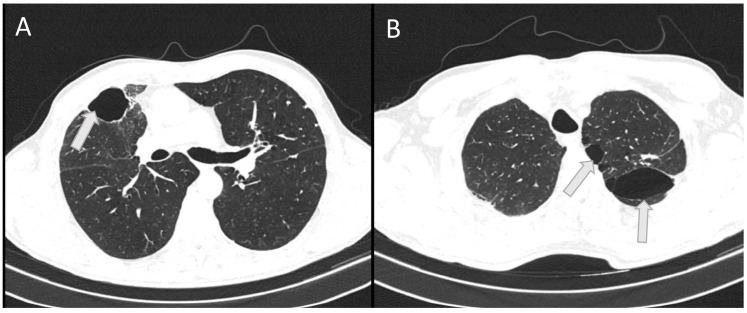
The horizontal CT scan section at the level of the 6th thoracic vertebra (A) and at the level of the 3rd thoracic vertebra (B) showing residual bullous changes (arrows) 6 months after severe chest injury with bilateral pneumonia in a 53-year-old patient.
